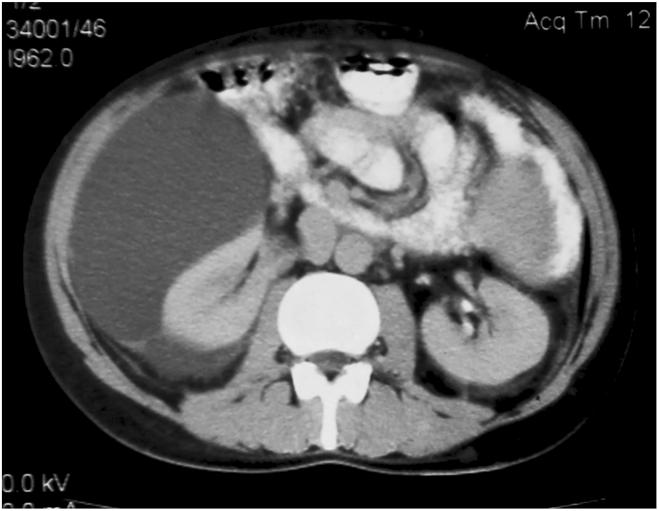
A contrast enhanced CT scan of the abdomen demonstrates showing a large right-sided perinephric fluid collection.
